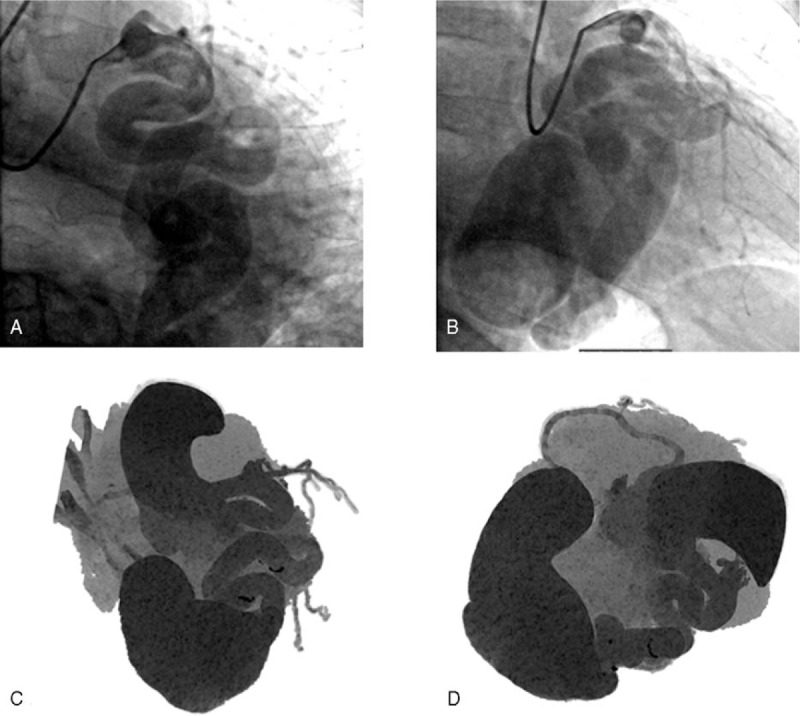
(A–B) Invasive coronary angiography and (C–D) Maximum intensity projection (MIP) cardiac computed tomography (CCT) images representing the fistulous tract.
